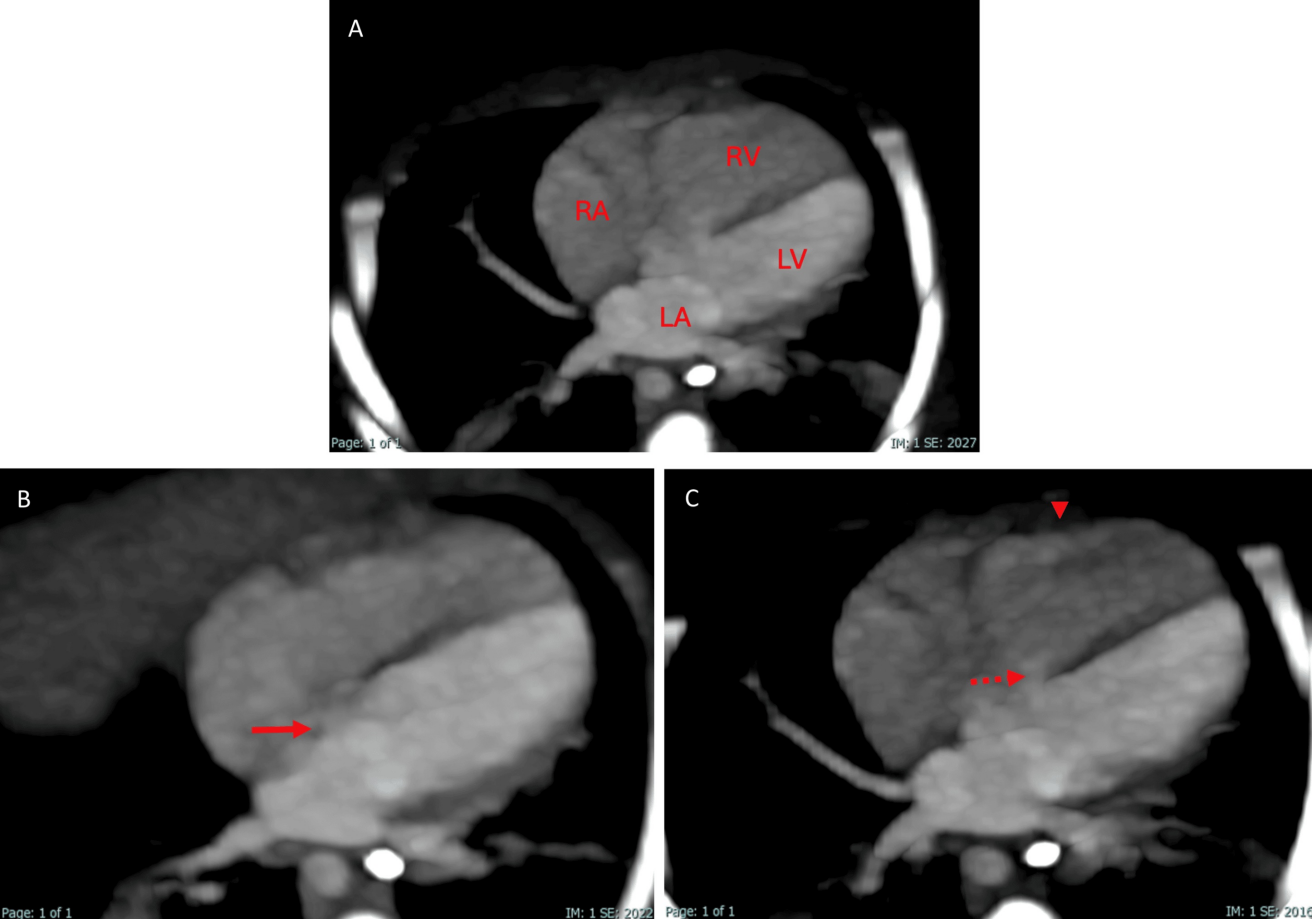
Cardiac CT angiography in oblique axial cuts at the level of the heart. (A) The image shows the right auricle (RA), left auricle (LA), right ventricle (RV), and left ventricle (LV). (B) At other levels of the plane, an atrial septal defect (arrow) is demonstrated. (C) Additionally, the ventricular septal defect (dashed arrow) and right ventricular hypertrophy (arrowhead) are observed.
